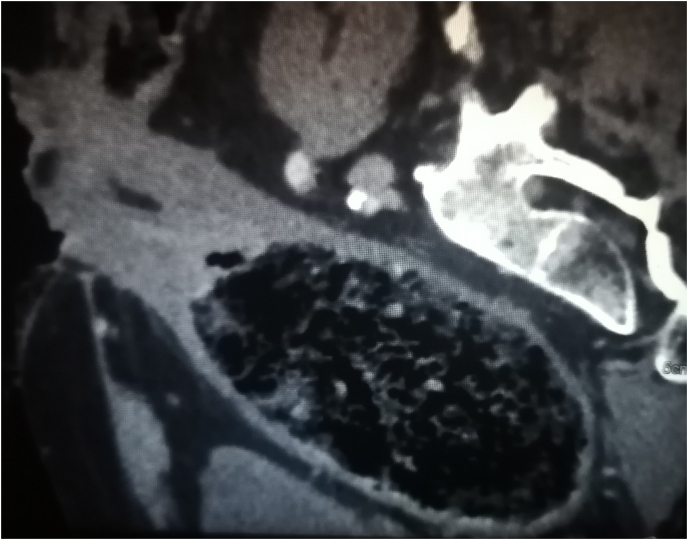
CT scan in the sagittal plane showing the megarectum after the first surgery.
